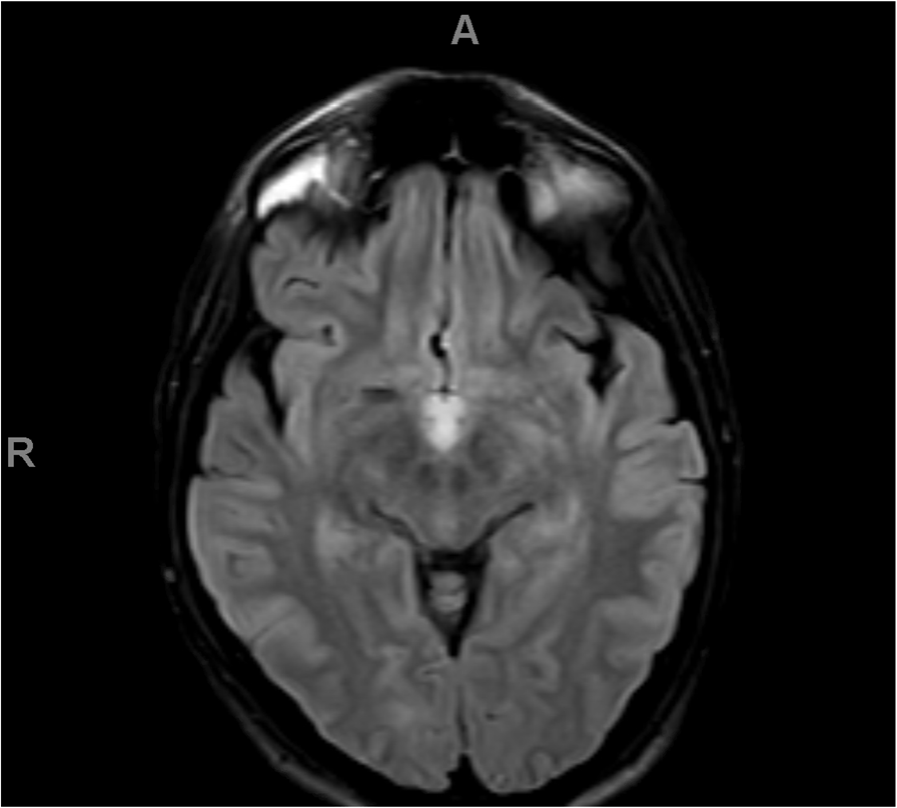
FLAIR axial images demonstrating high signal alterations in the mamillary bodies
